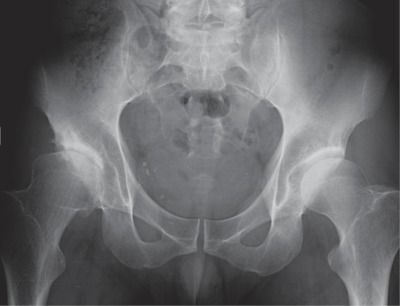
Long-term follow-up radiograph of a 44-year-old man where there was discrepancy between the observers in the assessment of the right hip. Using the minimum JSW method, observer 1 found no OA (a minimum JSW of 3.2 mm) whereas observer 2 found OA (a minimum JSW of 1.8 mm). Both observers found severe OA by Kellgren and Lawrence (grade 3) and Croft (grade 4).
